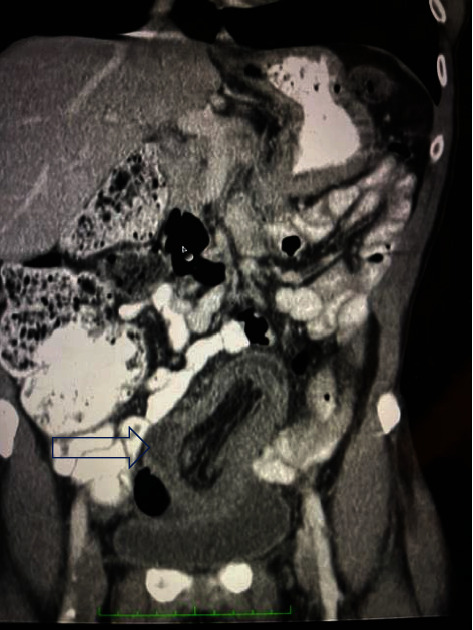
Coronal view of abdominal CT scan showing colorectal intussusception.
